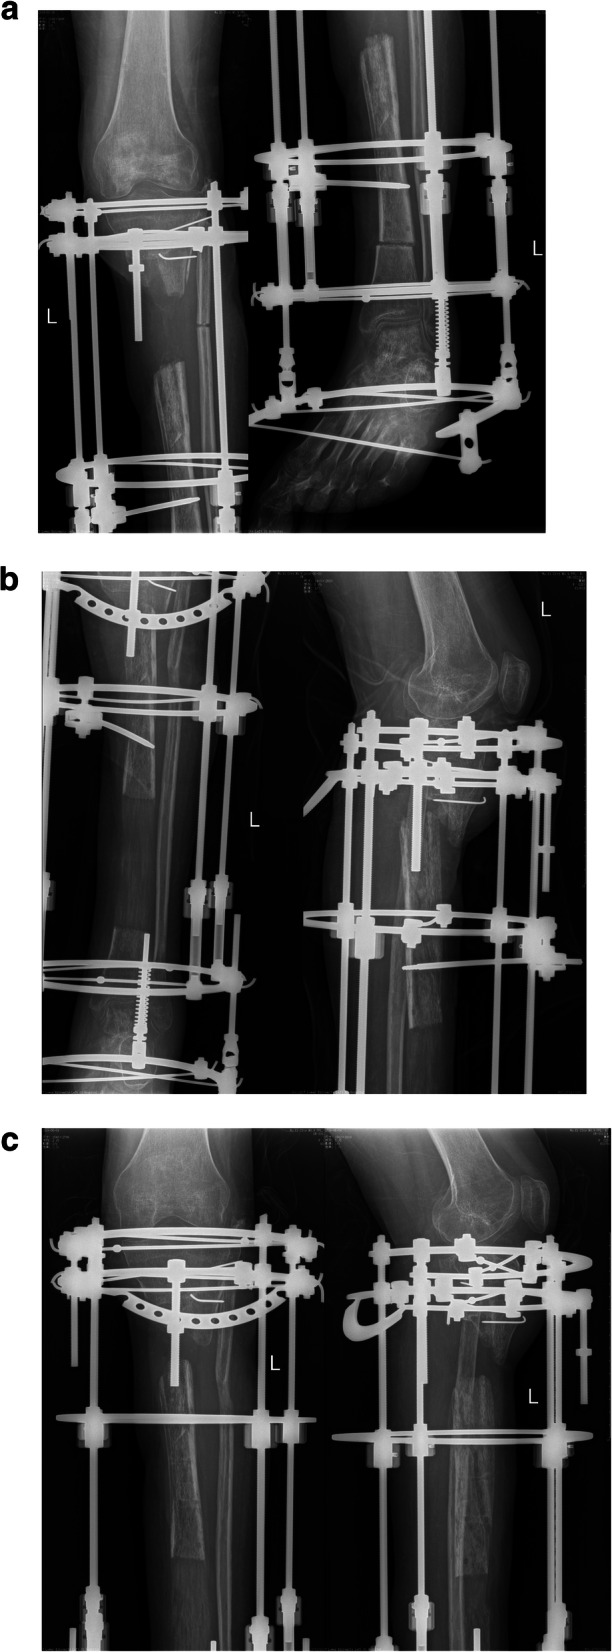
Patient with fracture and defect of tibia and fibula was treated by bone transport (a,b), in whom the X-Ray showed the TBS retracted 3.0 cm in a timing of removal of 3.5 months and a time interval of 25 days (c)
